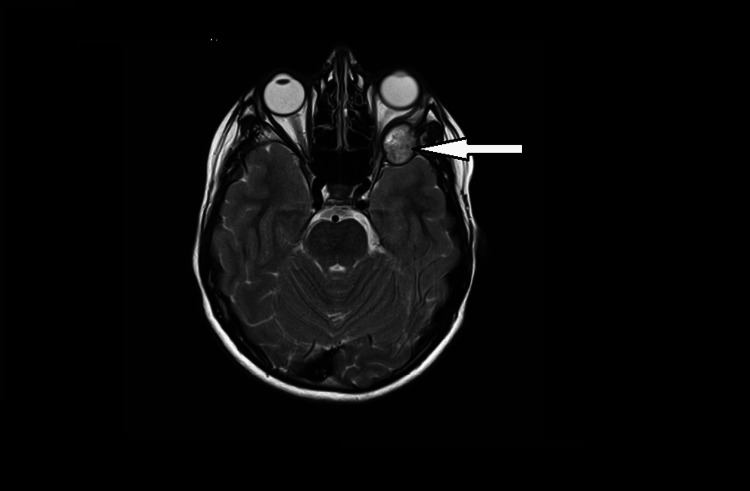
Axial MRI T2-weighted MRI imaging of the head The MRI shows a 2 cm lesion marked with an arrow.
